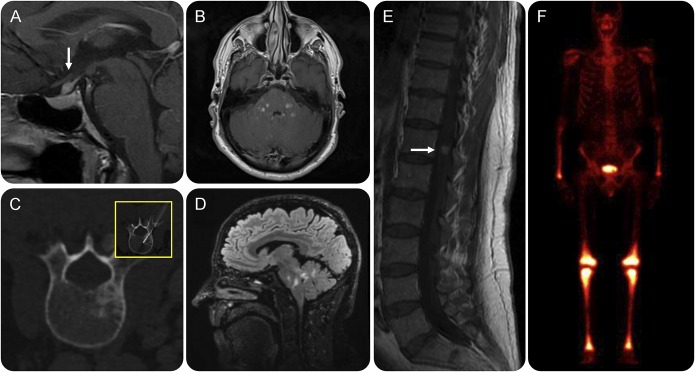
Clinical–radiologic diagnosis of Erdheim-Chester disease (A) Isolated infundibular lesion (arrow) causing diabetes insipidus at presentation. (B) Gadolinium-enhanced MRI scan of the brain; axial sections through pons show multiple enhancing lesions. (C) CT scan of spine shows isolated vertebral sclerotic lesion (biopsy shown was negative for lesional tissue). (D) Sagittal fluid-attenuated inversion recovery MRI of brain shows lesions distributed throughout the pons and cerebellum. (E) Enhancing lesion in cauda equina (white arrow). (F) Bone scan shows characteristic uptake in the long bones (femur, tibia).
